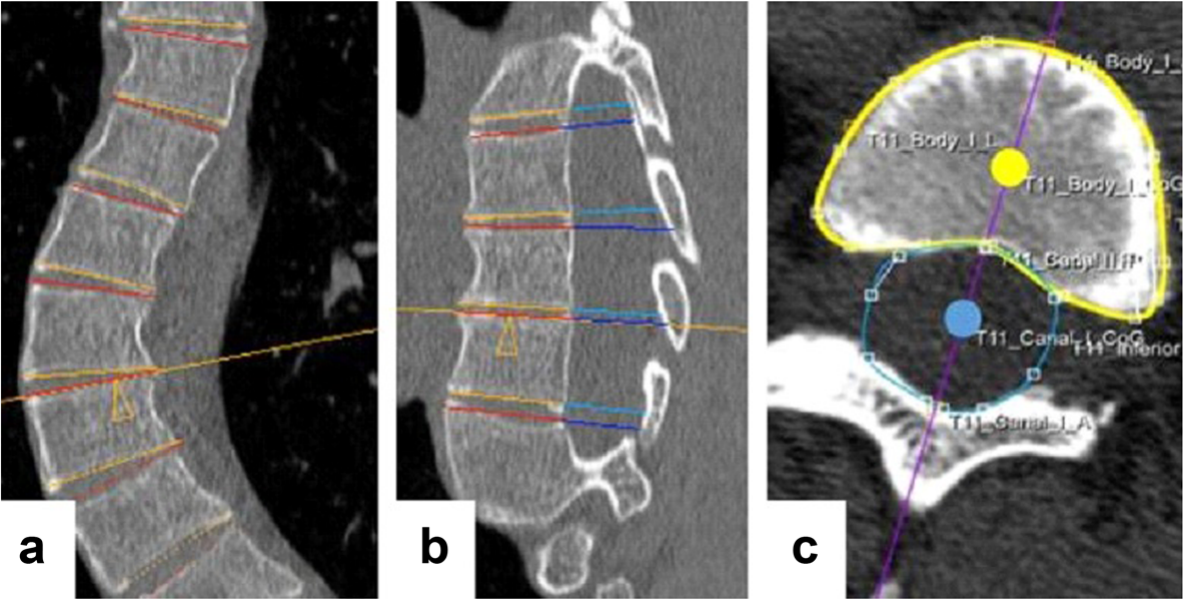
The orientation of the upper and lower endplates of each individual vertebra of the computed tomography scans was determined by using the semi-automatic software, correcting for coronal and sagittal (a and b) tilt, to reconstruct the true transverse sections. The observer drew a contour around the vertebral body (*yellow line* in c) and spinal canal (*blue line* in c). The software calculated a center of gravity of the vertebral body (*yellow dot* in c) and spinal canal (*blue dot* in c). For each endplate, its longitudinal axis was calculated as the line between those two points (*purple line* in c). The rotation of this axis minus the rotation of the neutral sacral plate represents the rotation of the endplate
